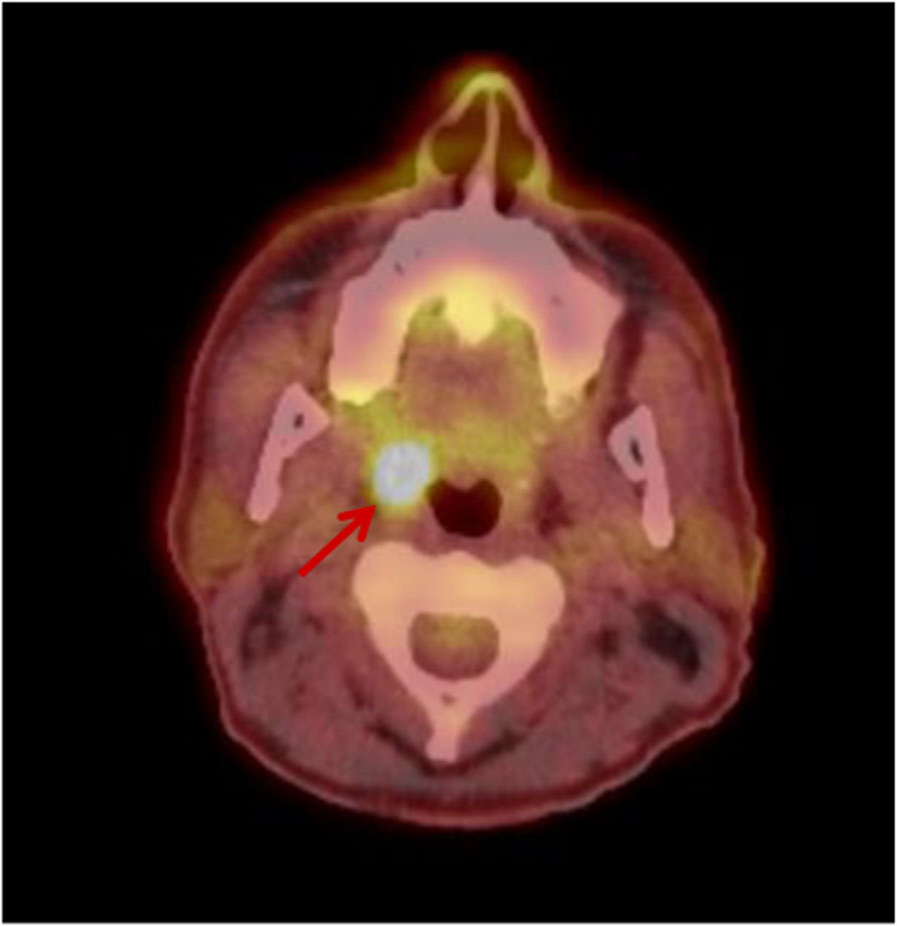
Axial PET-CT scan. An asymmetric contrast medium enhancement of the *right* tonsil is visible (*arrow*). No enhancement of the *left* tonsil
